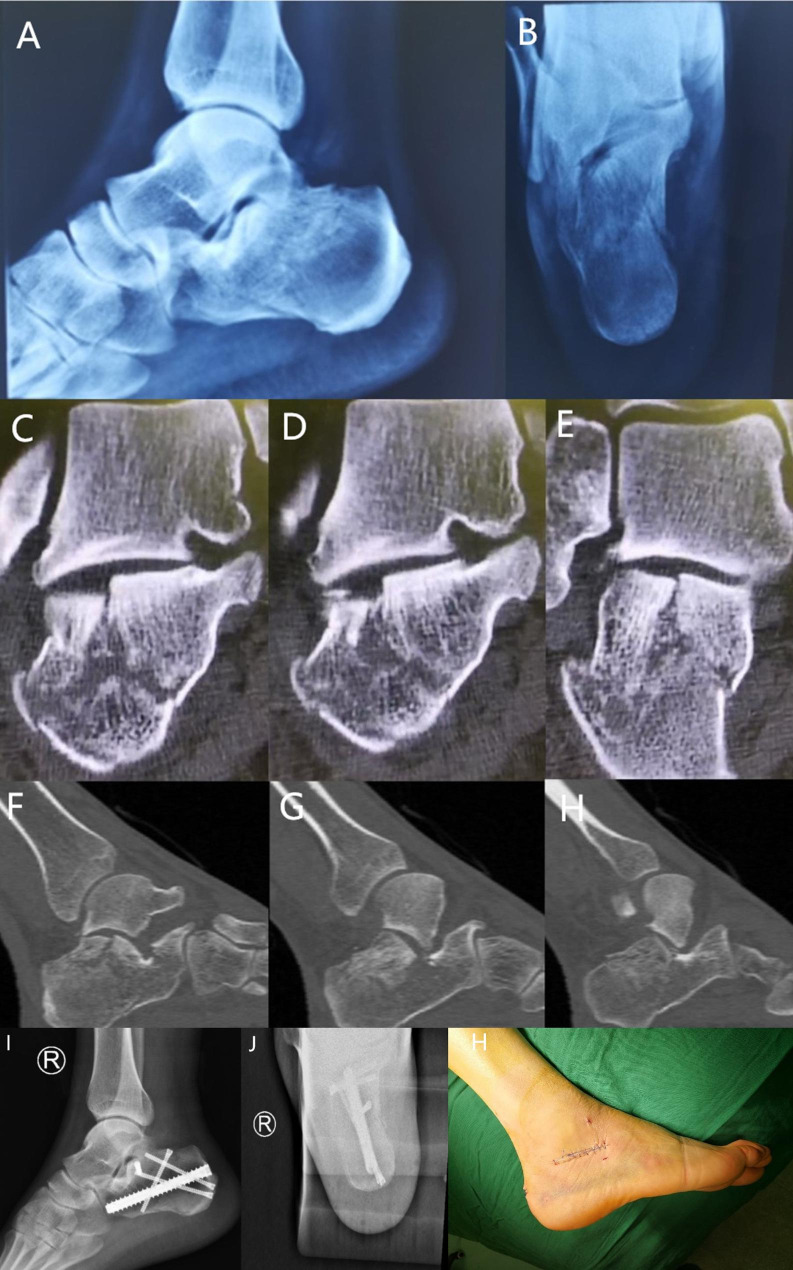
Case 1. Male, 39 years old, who fell from a ladder X-ray showed a calcaneous fracture. Later view (a) Axial view (b)
